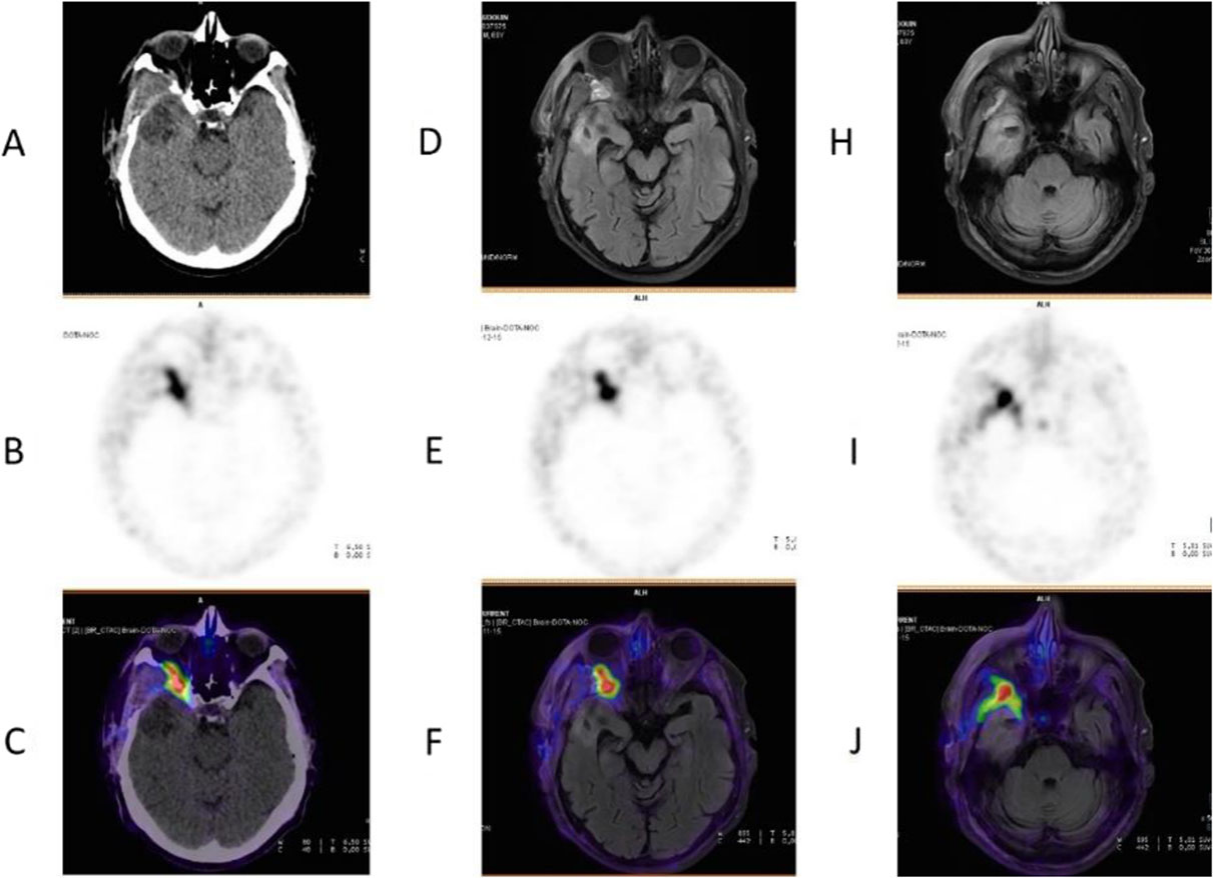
^68^Ga-DOTA peptide, meningioma. *Clinical history*: 70 y.o. man with meningioma of the skull basis. *PET/CT findings*: ^68^Ga-DOTATATE shows highly increased uptake in the right sphenoidal bone area with an extension to the orbital cavity, corresponding to the meningioma (a CT; d, h MRI; d, e, i PET; c fused PET/CT; f, j fused PET and MRI)
